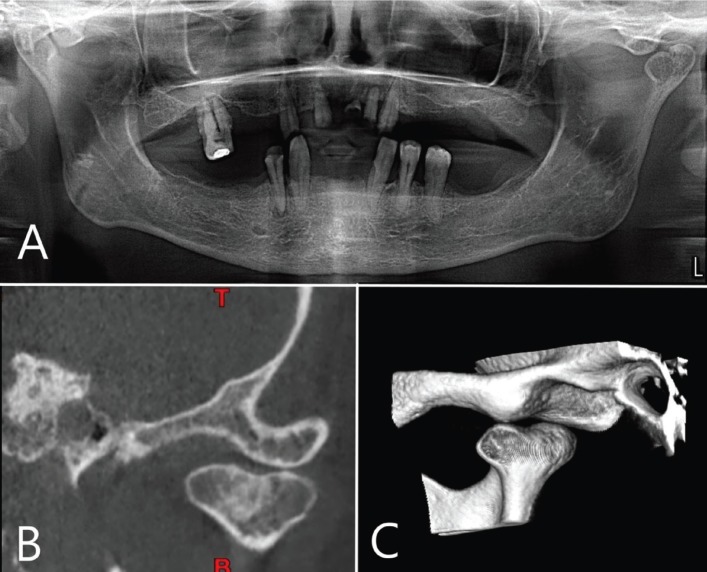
Case 1. A) Panoramic radiography shows a left BMC. B) Coronal slice from computed tomography. Mediolateral condylar position. C) 3D reconstruction. Posterolateral aspect.
